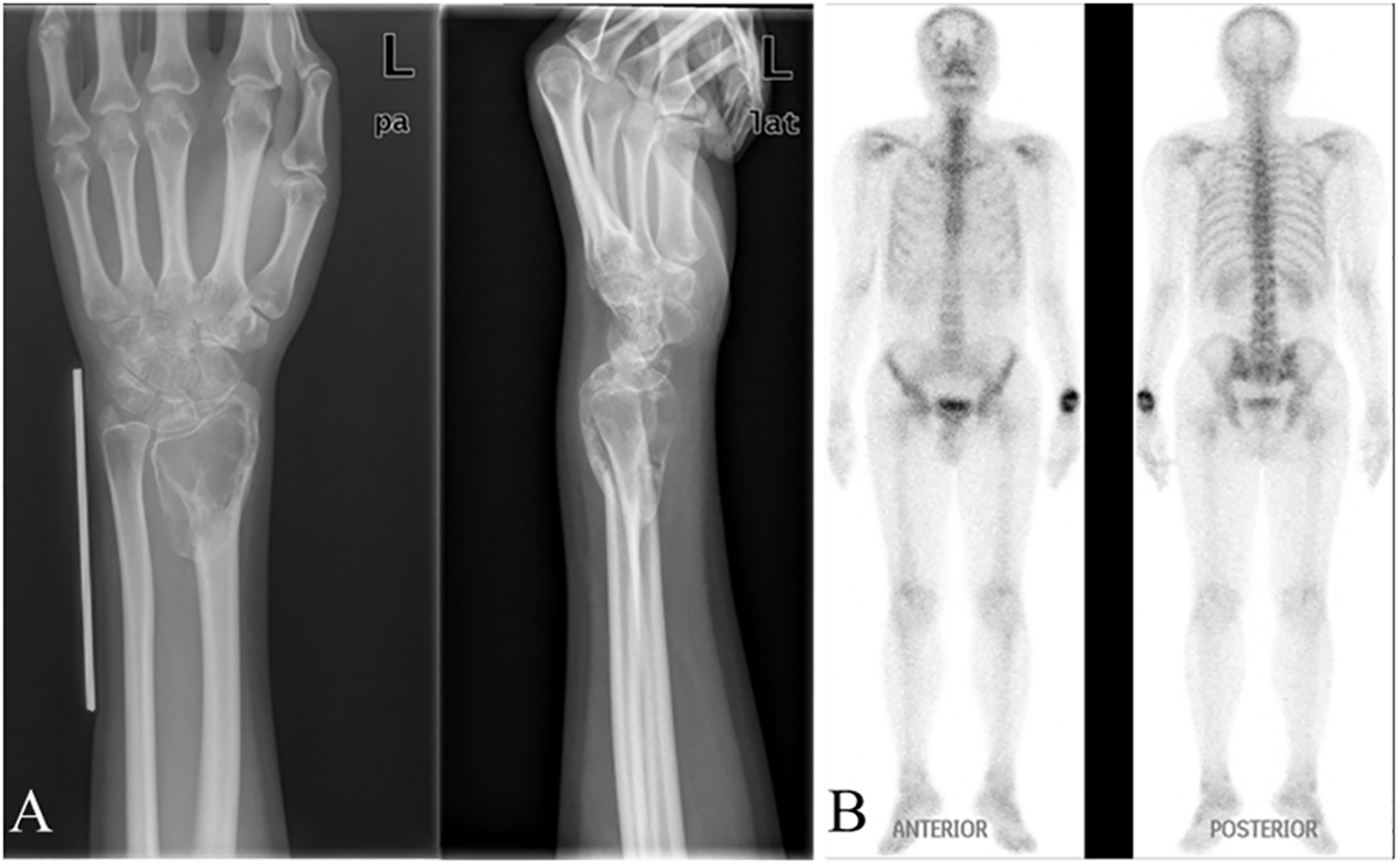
Preoperative examination results of a typical patient. A 20-year-old female patient diagnosed with a giant cell tumor of the distal left radius (Campanacci grade III), with preoperative (A) X-ray and (B) SPECT examination results.
